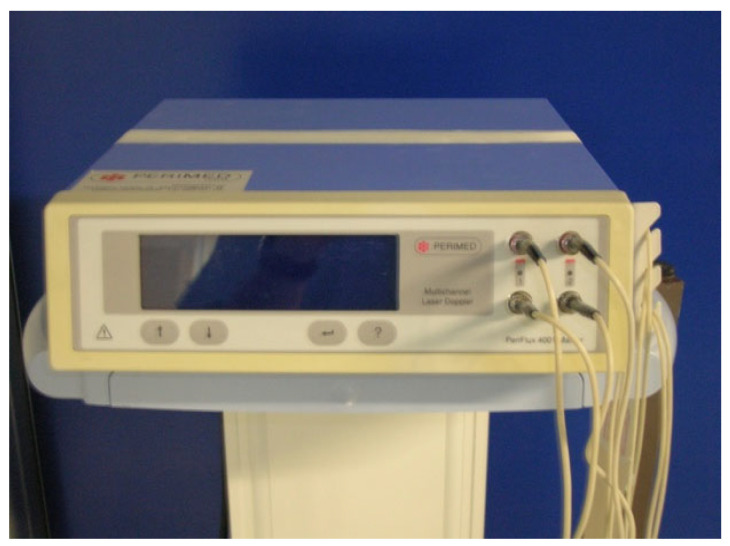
Laser Doppler fluxmeter.
